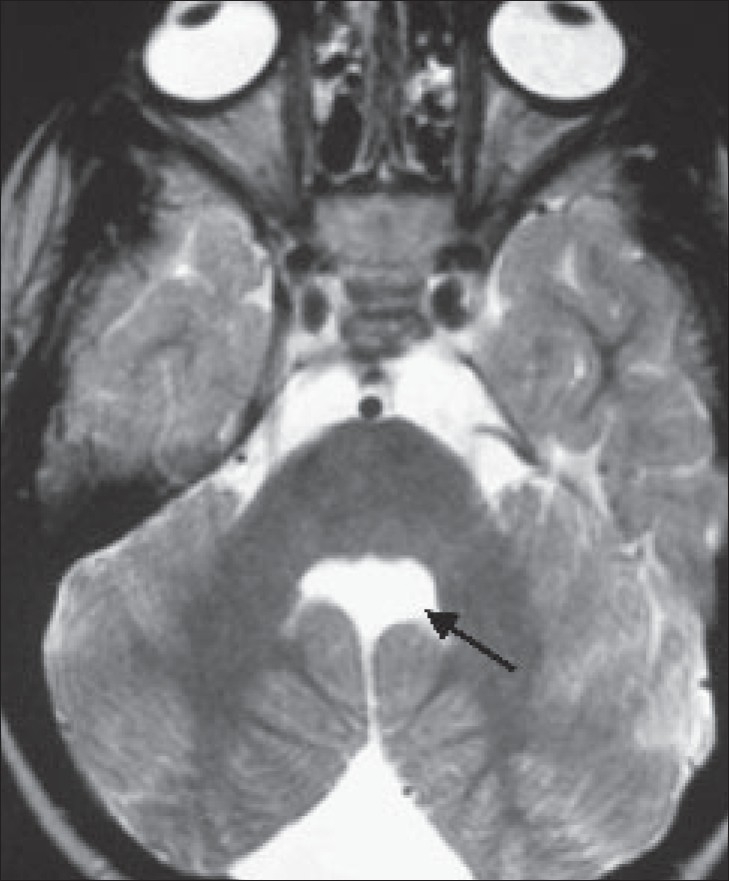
Axial T2W MRI of the brain shows the typical ‘bat-wing’ shaped fourth ventricle. The fourth ventricle is in direct contact with the extracerebellar subarachnoid space and the cerebellar hemispheres are in contact with each other
